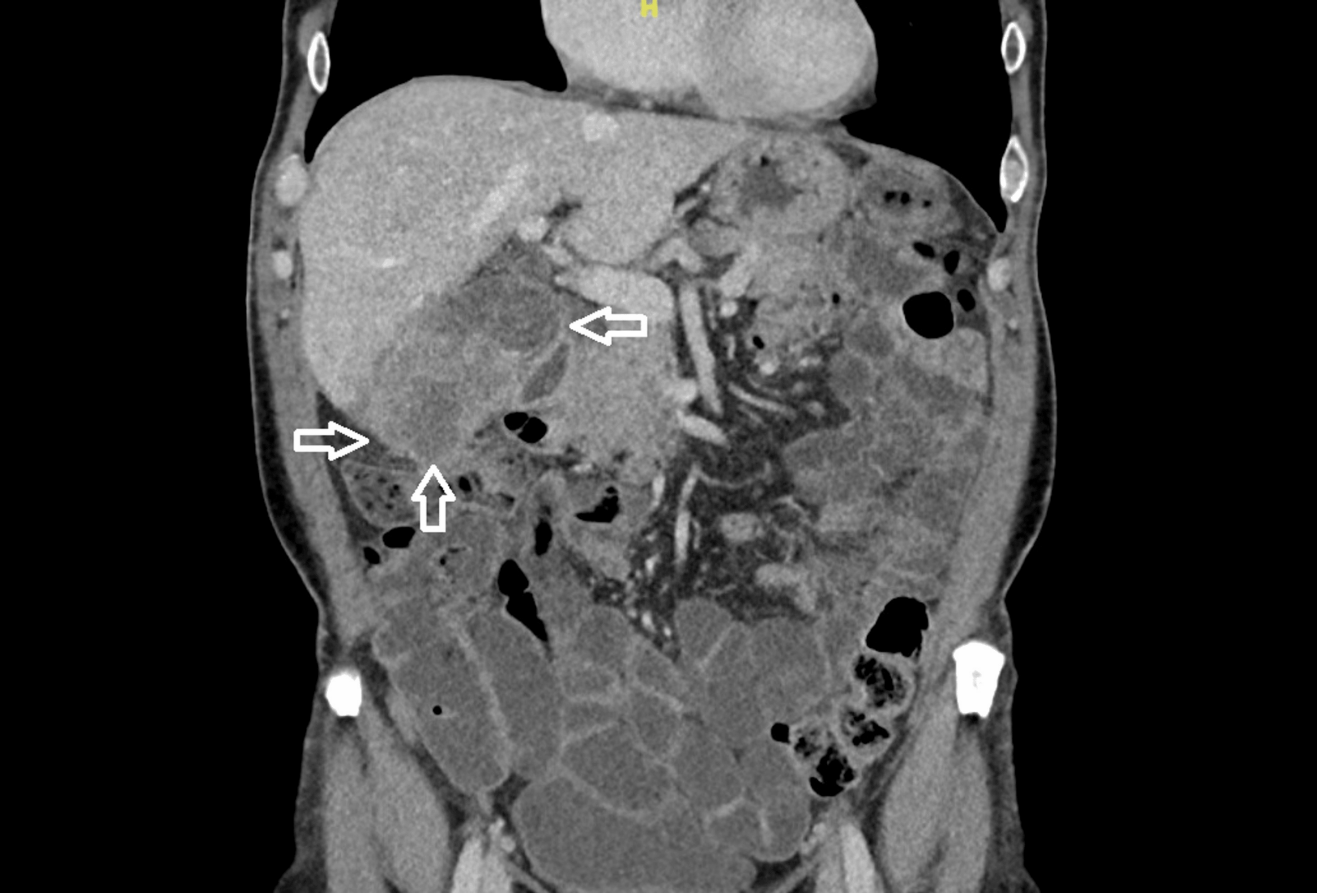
CT findings of the gallbladder tumor in sagittal view. The arrows point out the tumor mass. CT: computed tomography
